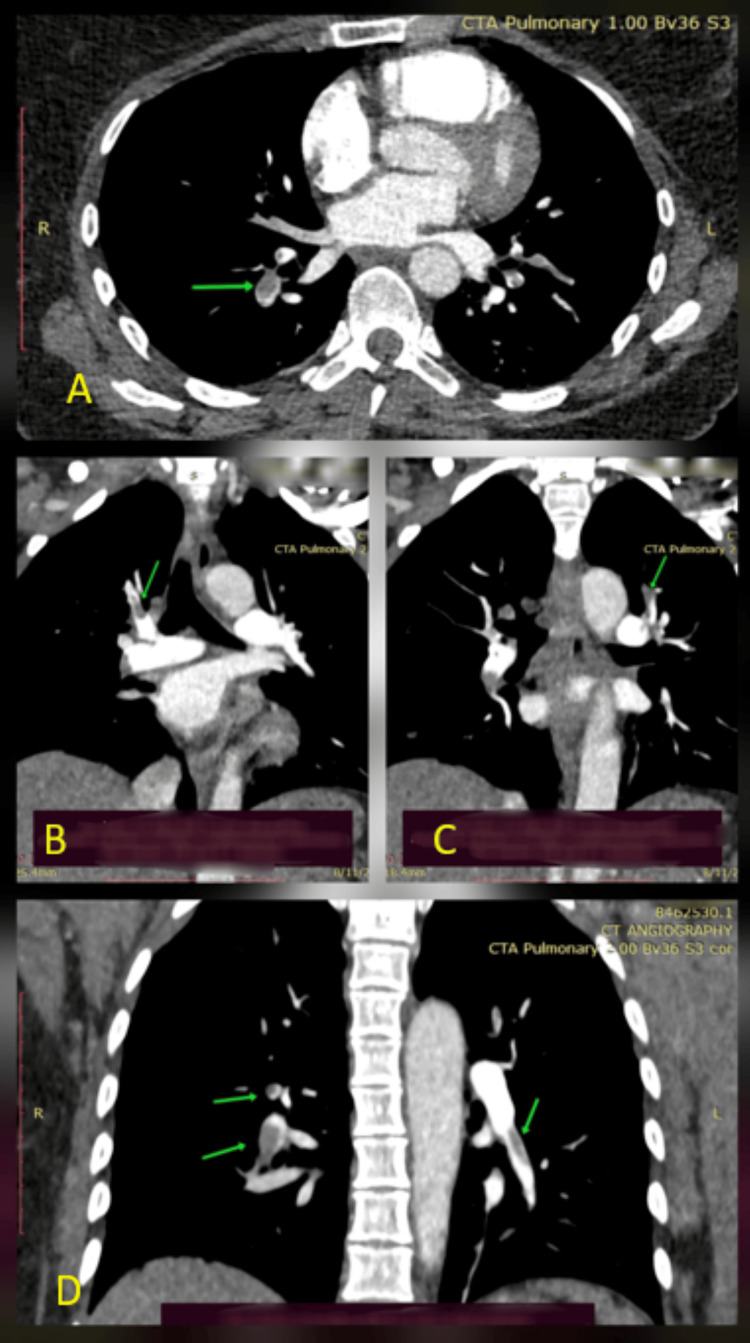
Computed tomography angiography images demonstrating pulmonary embolism (arrows): (A) axial view, (B) coronal view, (C) coronal view, and (D) coronal view.
